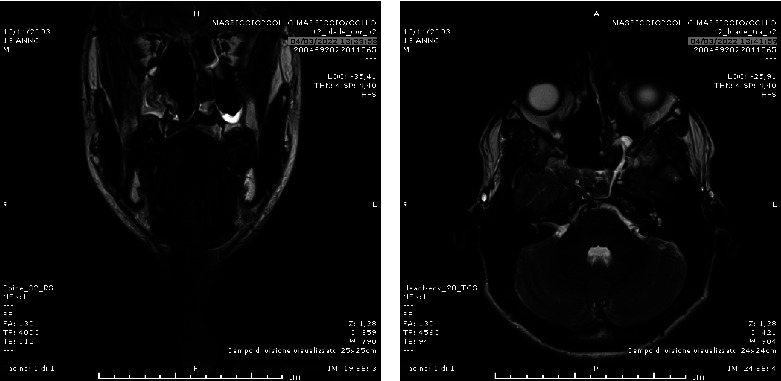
Postprocedural MRI scan performed at 1 month after surgery: coronal (a) and axial (b) T2-weighted views which show the complete resection of the JNA with empty nasal left fossa, with parietal reactive mucous thickness.
